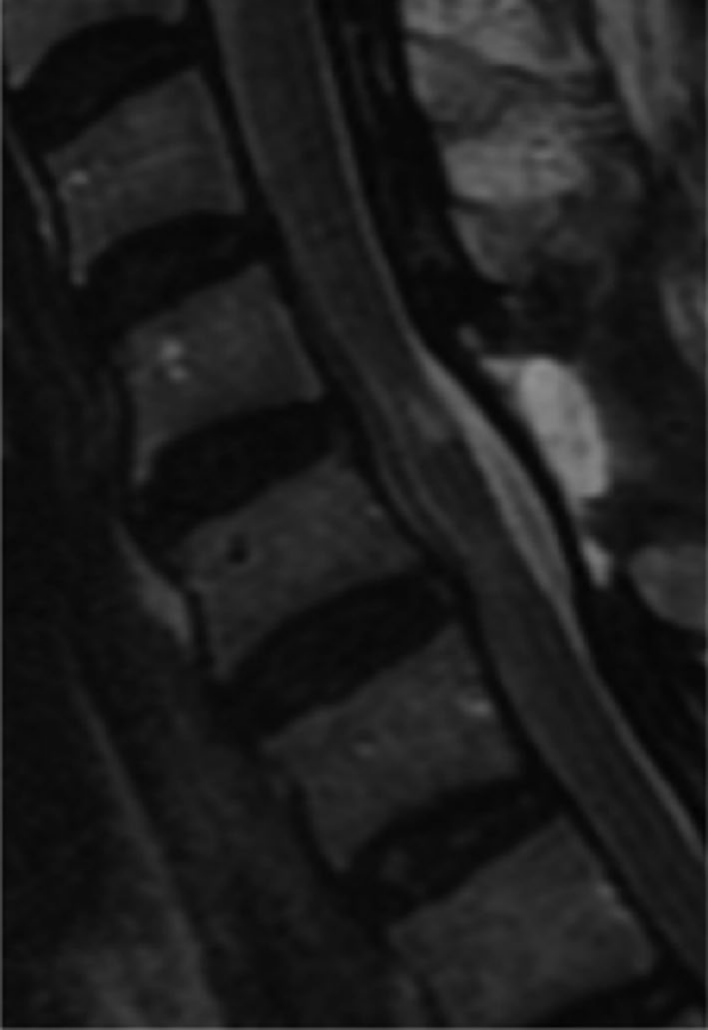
Sagittal MRI in T2-weighted sequence showing decompression of the spinal cord after laminectomy
